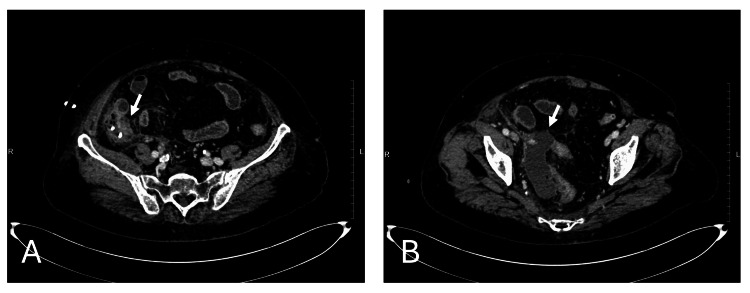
Contrast-enhanced CT findings on hospital day 2. (A) The pigtail catheter remained within the abscess cavity; however, the abscess had not decreased in size. A high-density foreign body suspected to be an endoscopic clip was still present within the abscess cavity (arrow). (B) Increased pelvic ascites and newly developed peritoneal thickening (arrow) suggested worsening peritonitis.
